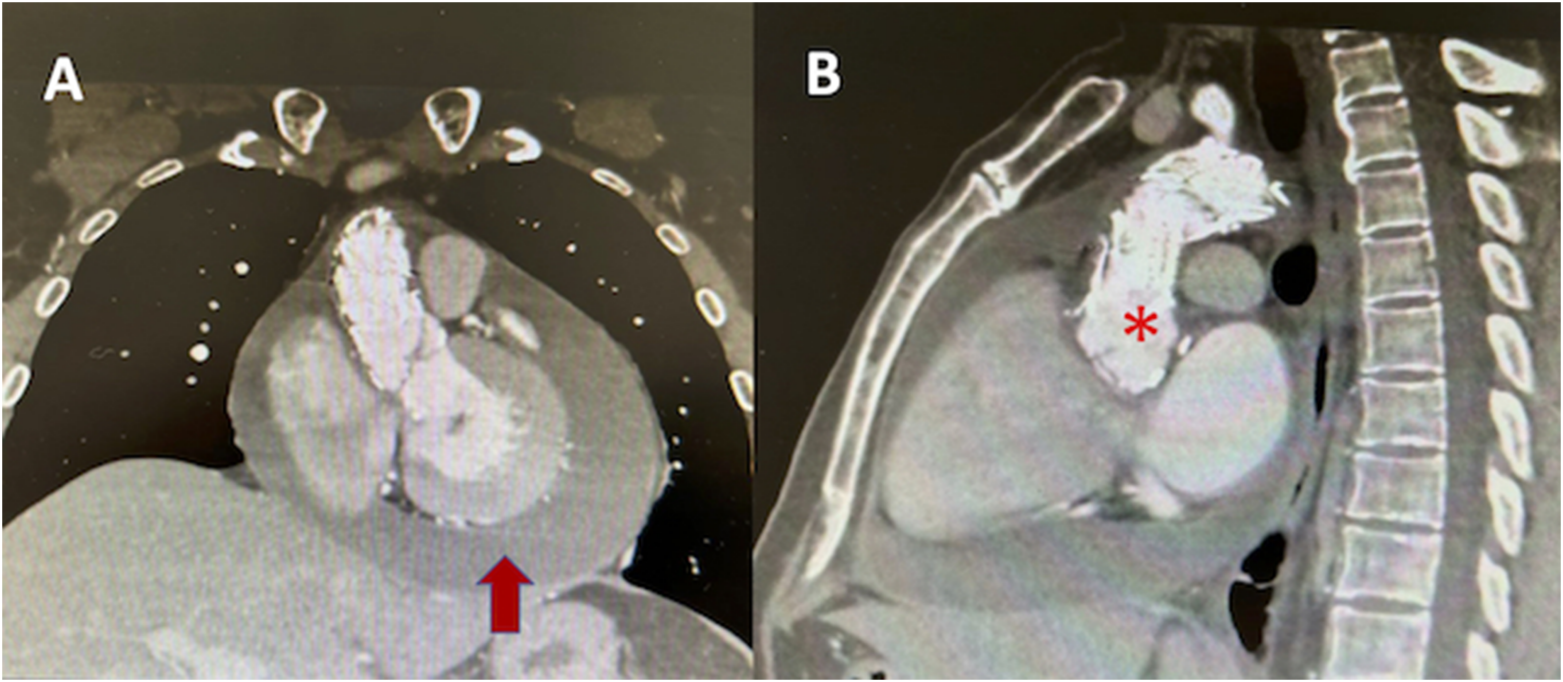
Angio CT of aorta (A) coronal axis showing pericardial effusion (red arrow), (B) endovascular material in ascending aorta (asterix).
